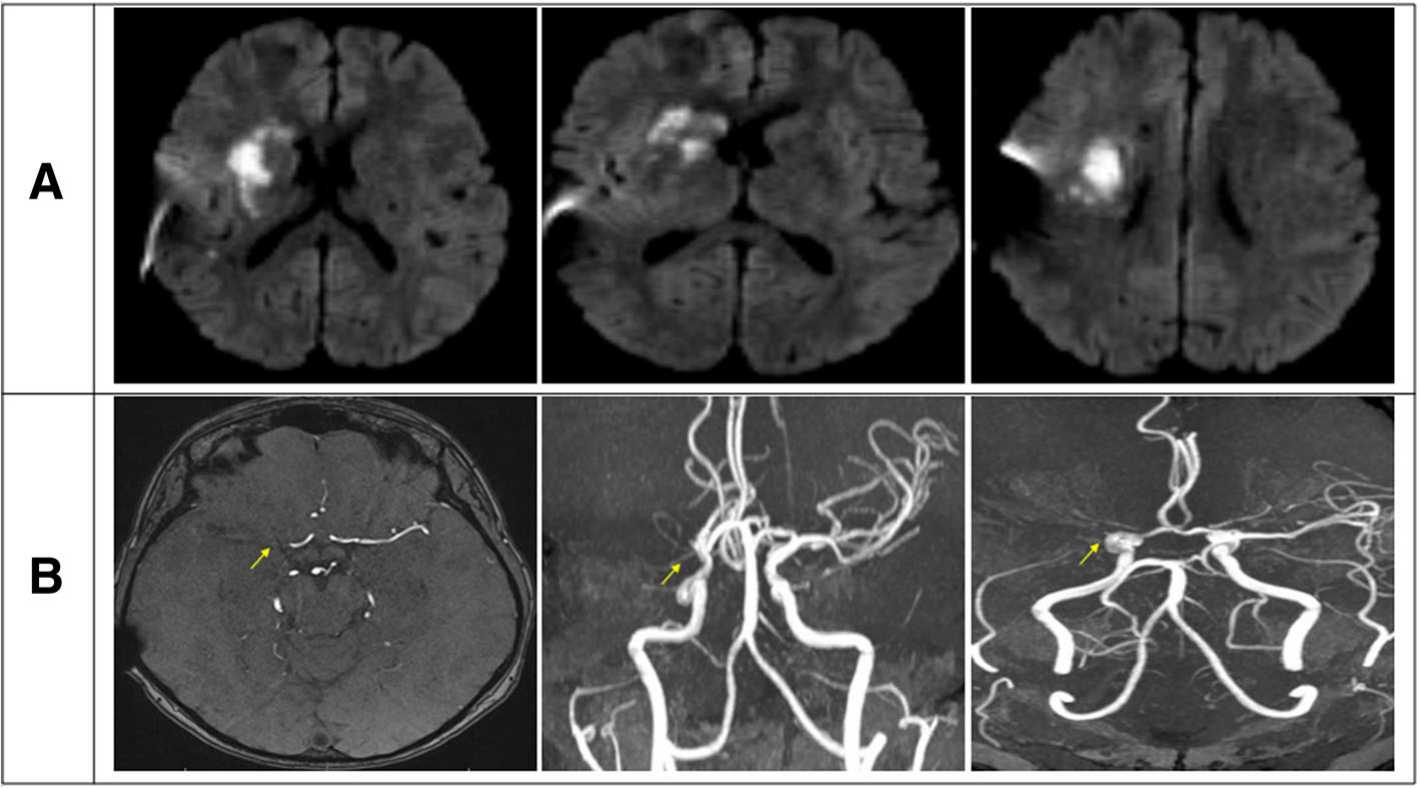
a Diffusion-weighted magnetic resonance imaging of the brain showing acute infarct centered in the right basal ganglia, internal capsule, external capsule, and corona radiata. b Magnetic resonance angiogram of circle of Willis showing complete occlusion of the right middle cerebral artery origin (*yellow arrows*)
